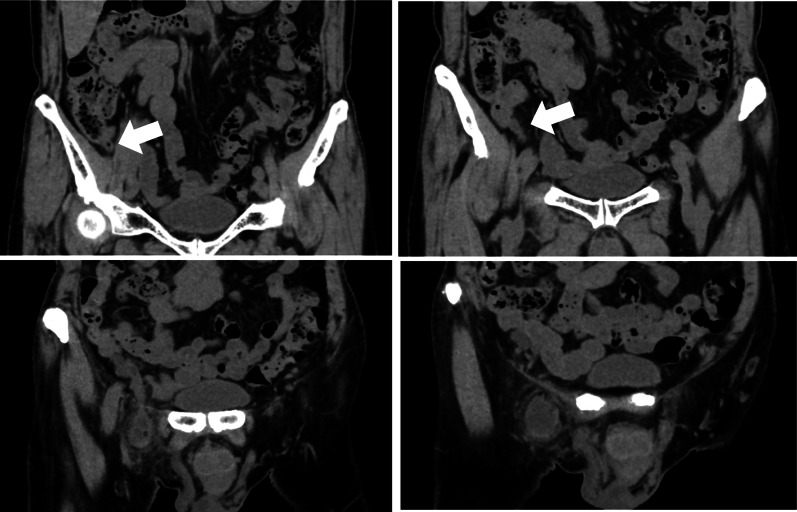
A CT scan of the abdomen shows a right femoral hernia in which the appendix is prolapsed. A mass-like accumulation of fluid was observed around the appendix in the hernia contents
